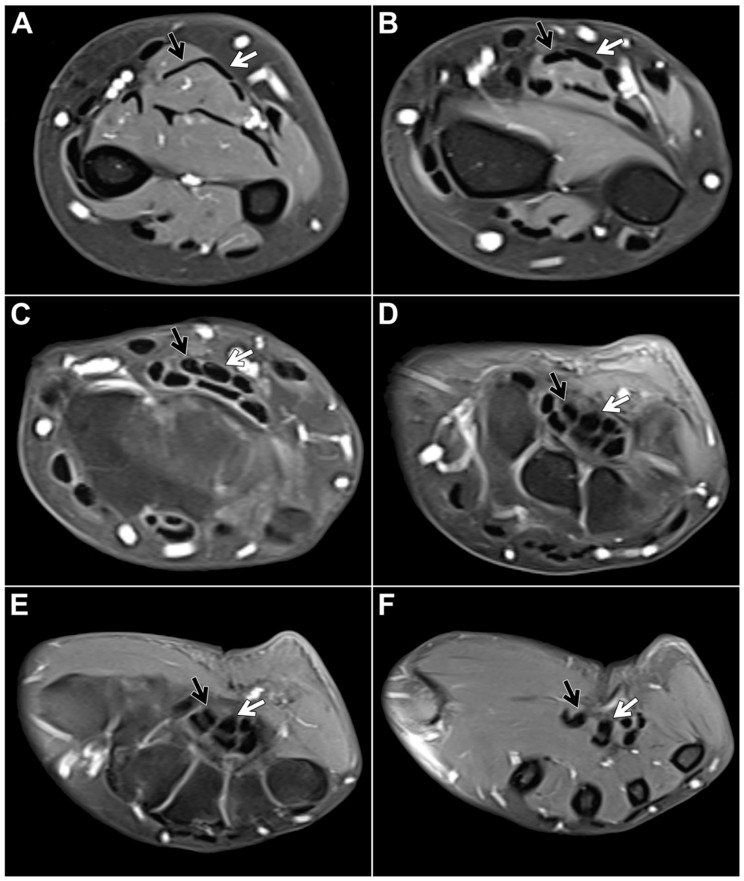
Contrast-enhanced, fat-suppressed T1-weighted turbo spin echo in a 44-year-old woman for presumed inflammatory disease of the right forearm depicts an anatomical variant of FDS anatomy without a typical (distal) chiasma antebrachii. Instead of an intersection, the distal FDS/2 (black arrow) and FDS/3 (white arrow) tendons run parallel to each other ((A,B): forearm level, (C–E): carpal tunnel level, (F): midcarpal level).
